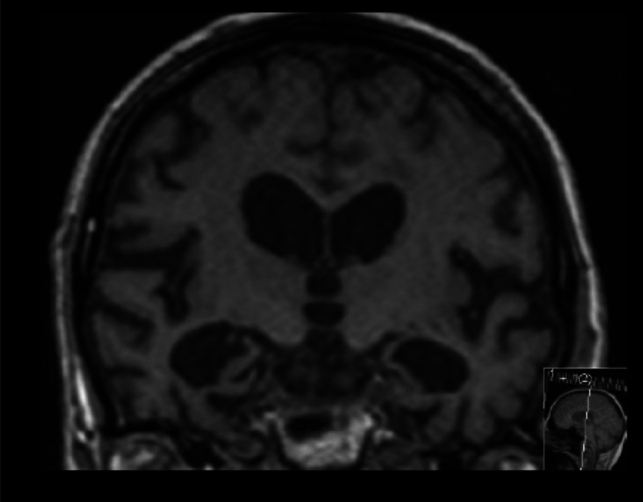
An 86-year-old patient presents with an MMSE score of 25/30 and APOE ε3/3. Cerebrospinal fluid analysis reveals no amyloid or tau pathology. MRI shows severe atrophy in both hippocampi, graded as MTA stage 4*, while cortical atrophy is minimal and notably disproportionate to the hippocampal changes. This presentation strongly resembles the clinical and pathological features associated with “pure” LATE-NC. *MTA (medial temporal lobe atrophy) visually rated by a semiquantitative scale (Scheltens et al. 1992) ranging from 0 to 4, where 4 stand for end stage hippocampal atrophy
